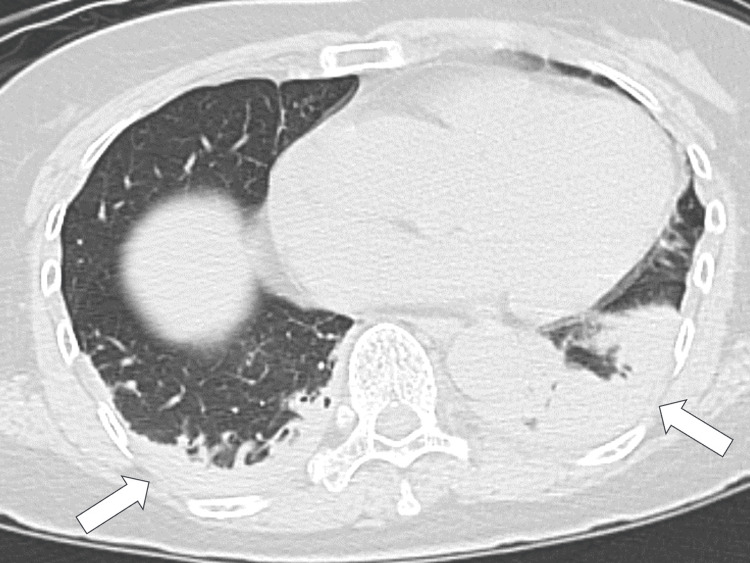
Chest computed tomography revealing bilateral infiltrations on the chest, indicating bilateral persistent pneumonia (white arrows).
